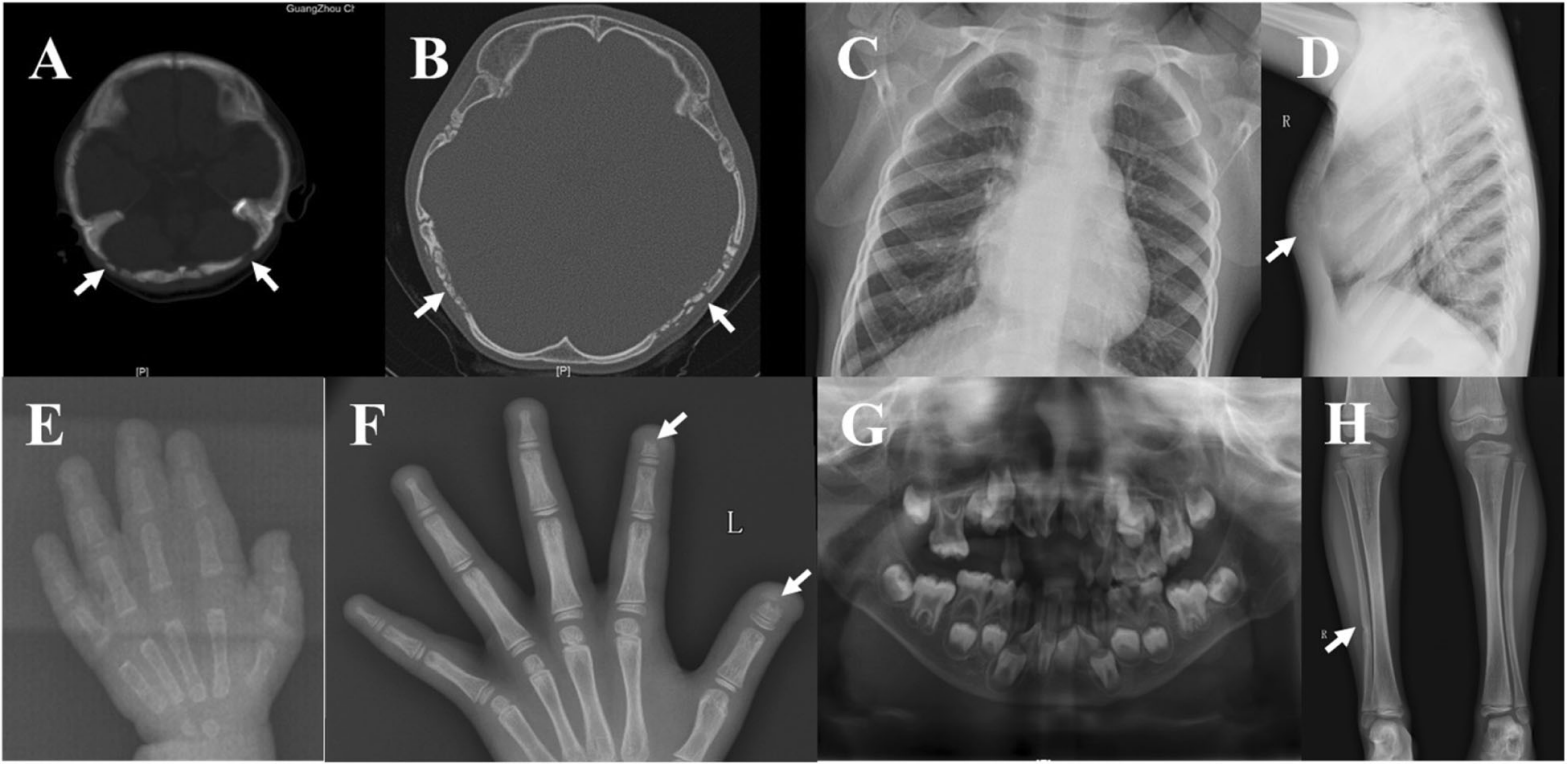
Computed Tomography (CT) scan and radiographs. a and b Wormian bones (arrow) and patent cranial sutures at 1 year and 2 months of age and at 7 years of age, respectively; c and d Wormian bones and deformity of the sternum at 7 years of age (arrow); e and f Acro-osteolysis was not present at 1 year and 2 months of age but was present in the distal phalanges at 7 years of age (arrow); g Misaligned and abnormally shaped teeth with dental malocclusion at 7 years of age; h Radiographs exhibiting bowed fibulae at 7 years of age (arrow)
